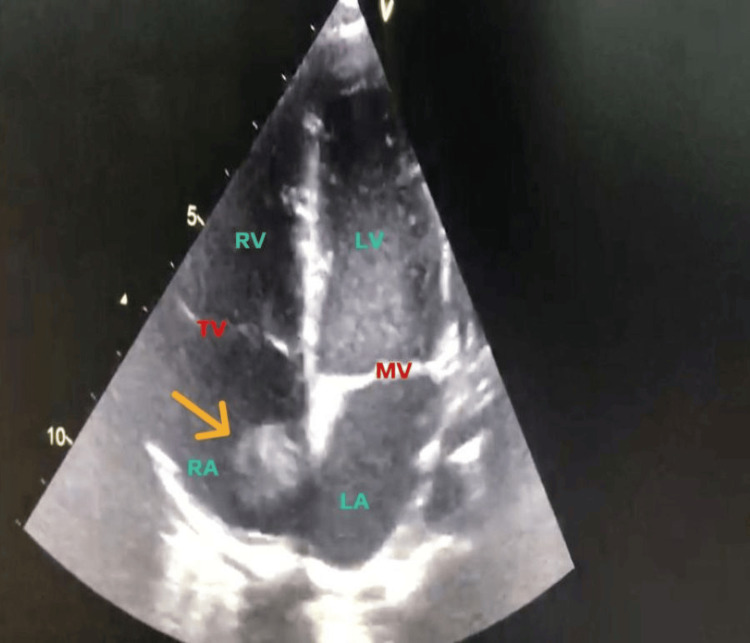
2D echocardiography showing a right atrial thrombus (arrow). RA: right atrium; LA: left atrium; RV: right ventricle; LV: left ventricle; TV: tricuspid valve; MV: mitral valve
